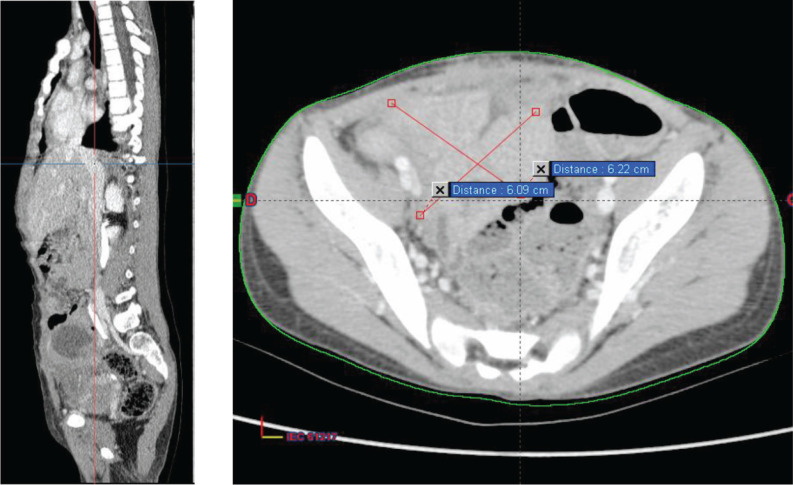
Chest–abdomen–pelvis postchemo and preoperative CT scan showed regression of the abdominal–pelvic tumour process estimated at 70%.
